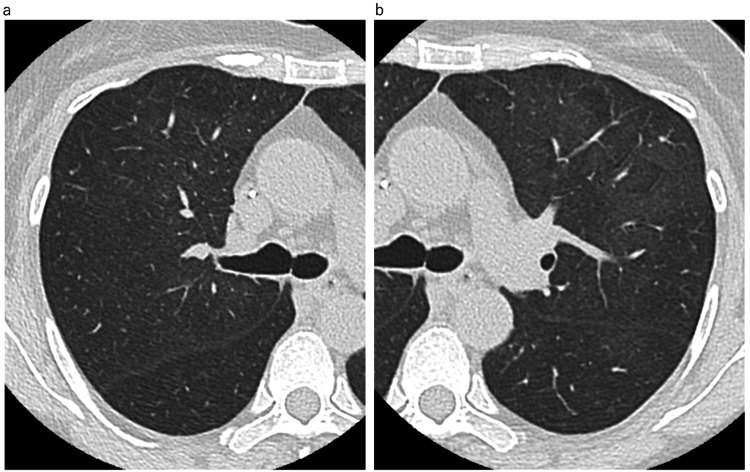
High-resolution computed tomography (HRCT) of Case 3 on admission a,b: HRCT showing slight bilateral diffuse ground-glass opacity.
